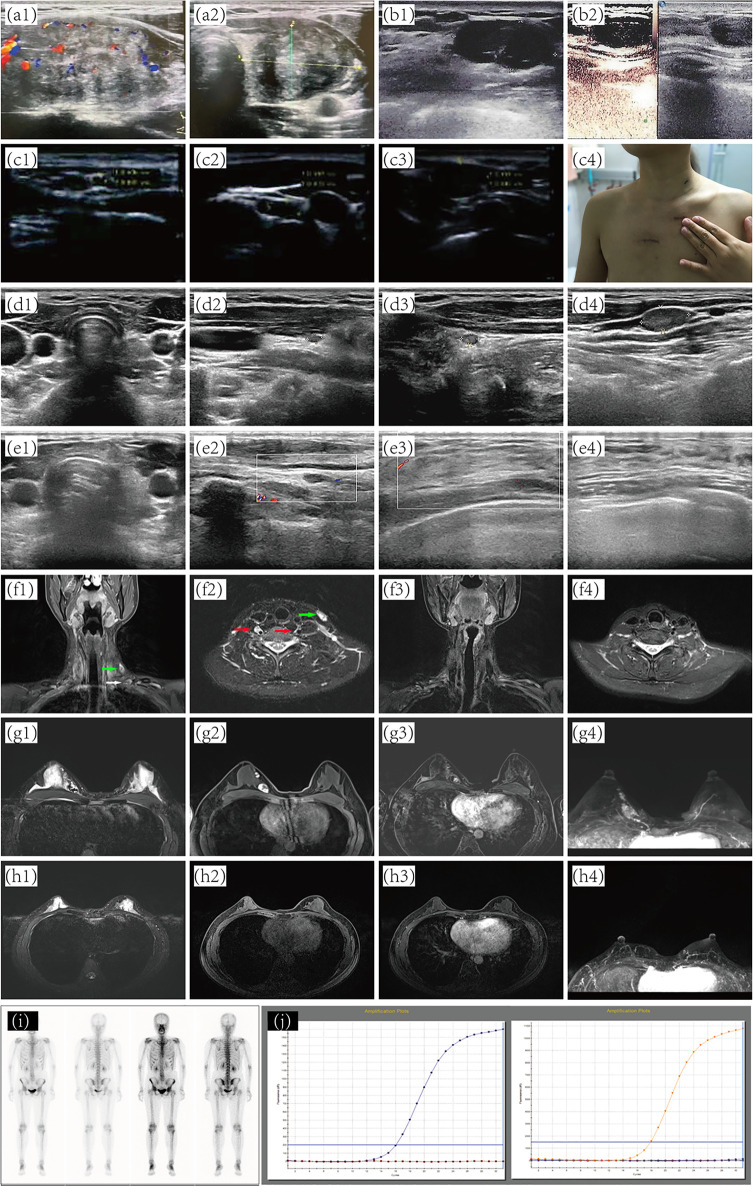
Preoperative and postoperative imaging diagnosis. (A) preoperative thyroid sonography. (B) Subcutaneous nodules around the right areola of the breast. (C) separate nodules in the right chest wall. (D) Ultrasound assessment of thyroid and cervical lymph nodes after patient receiving ETC. Ultrasound of thyroid (E1, 2) and breast (E3, 4) at five years post-operation. (F1, 2) metastatic lymph nodes. (F3, 4) No abnormality was found at five years post-operation. (G) A predominantly cystic mass with solid portions. No abnormality was found on MRI (H) and ^131^I-whole-body scan (I) at five years post-operation. (J) NRAS, BRAF, and PIK3CA genes by quantitative real-time PCR. ETC, Endoscopic thyroidectomy; MRI, magnetic resonance imaging.
